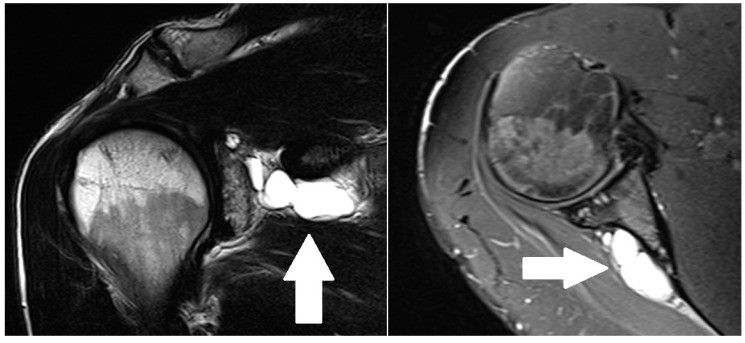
Patient affected by paralabral cyst of the right shoulder: coronal (left image) and transverse (right image) views of an MRI with contrast agent. The arrows highlight the cyst.
